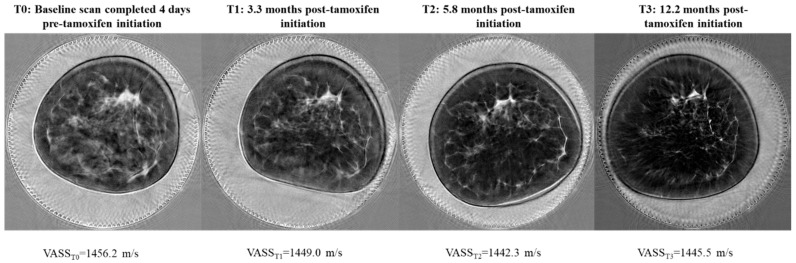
Example of single ultrasound tomography sound speed images from the breast contralateral to a diagnosis of invasive breast cancer in a tamoxifen-treated premenopausal patient over the course of the study follow-up period. Dense tissue appears white on the image with higher sound speed values, while fatty tissue appears dark with lower sound speed values. Among the tamoxifen-treated group, we observed significant VASS declines as early as 4–6 months post tamoxifen initiation (T2), with the strongest results observed among premenopausal patients.
